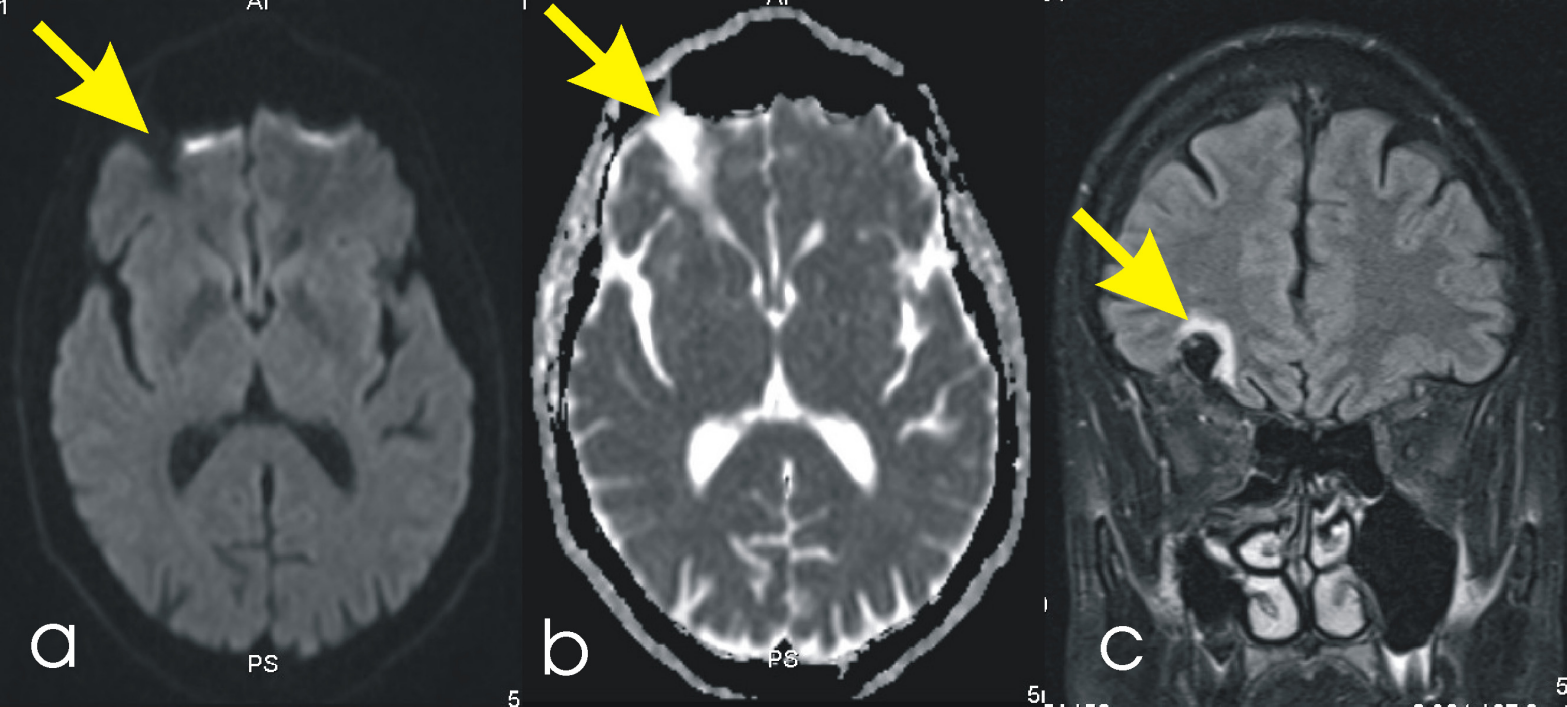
Exemplary slides of the sequences used to detect brain lesions. (A) Axial diffusion-weighted imaging, b1,000. The arrow points to an older postcontusional brain lesion. (B) Axial apparent diffusion coefficient map. The arrow points to the same lesion as in (A). (C) Coronal fluid attenuated inversion recovery (FLAIR) image. The arrow points to the same lesion as in (A).
